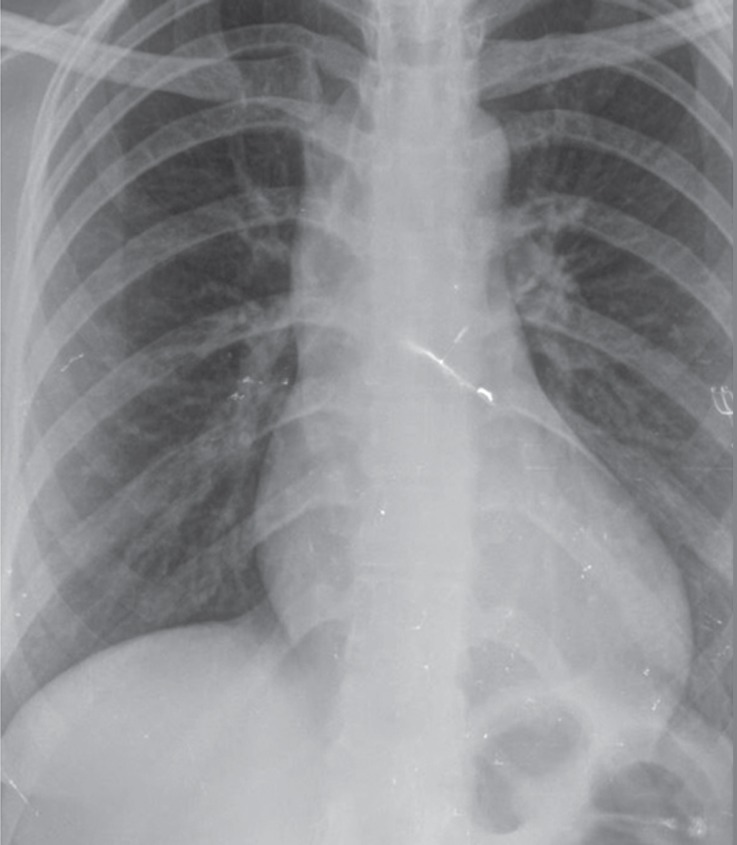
Chest X-ray (posteroanterior view) of the patient, depicting cardiomegaly.
